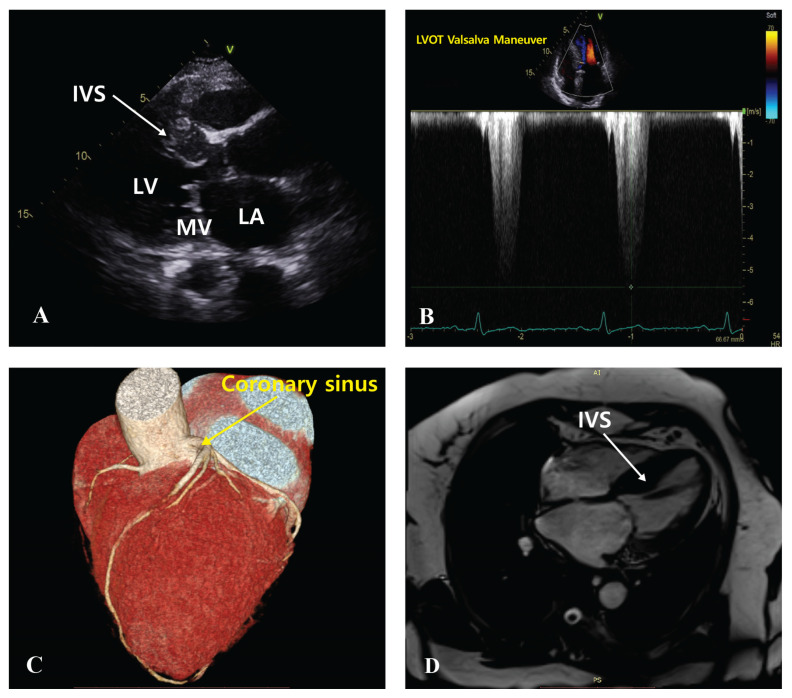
Imaging of IVS and LVOT using echocardiogram, CT, and MRI (pre-procedure). (A) Echocardiographic image showing the interventricular septum (IVS) with white arrows. (B) Echocardiographic image showing the measurement of the LVOT gradient during a Valsalva maneuver. (C) A 3D snapshot from CT imaging showing the anatomical structure of the coronary sinus. (D) MRI image showing the baseline IVS.
